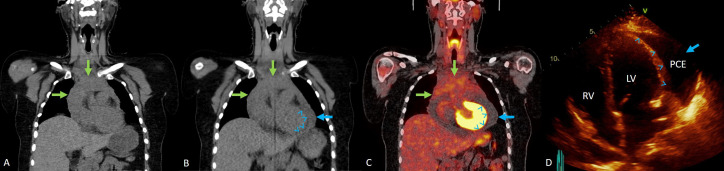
Coronal CT scan for proton therapy planning (A) shows residual enlarged mediastinal lymph nodes (green arrows). No pericardial effusion is noted. Coronal CT proton therapy fraction 8 (B) shows residual enlarged mediastinal lymph nodes (green arrows), and a new pericardial effusion (blue arrow heads and blue arrow). FDG-18 PET-CT fusion image after proton therapy fraction 8 (C) demonstrate FDG uptake in mediastinal lymph nodes (green arrows) consistent with a Deauville 3 response. No FDG uptake in pericardia effusion is demonstrated (blue arrow heads and blue arrow). Echocardiogram (D) confirms pericardial effusion (blue arrow heads and blue arrow) prompting patient intervention with pericardial window. Abbreviations: CT, computed tomography; FDG, fluorodeoxyglucose; PET, positron emission tomography.
